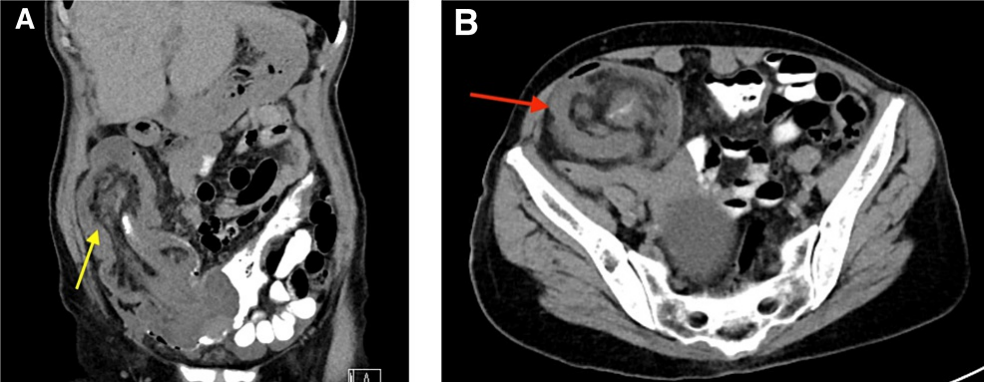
(A) Coronal view of the abdominal CT scan demonstrating ileocolic intussusception in the RLQ (yellow arrow). (B) Axial section showing the apple core appearance typical of Intussusception (red arrow).
